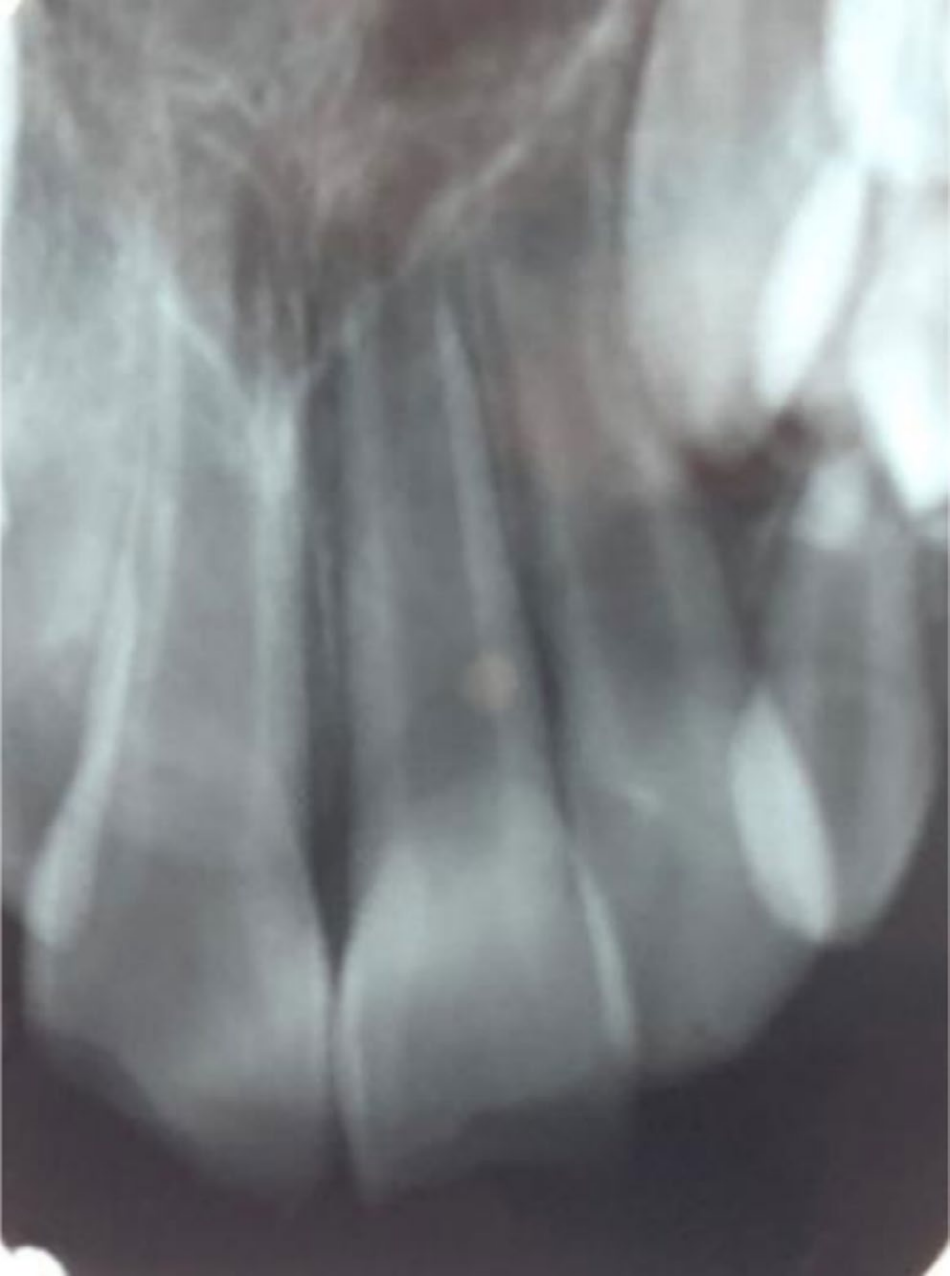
Preoperative radiograph.
